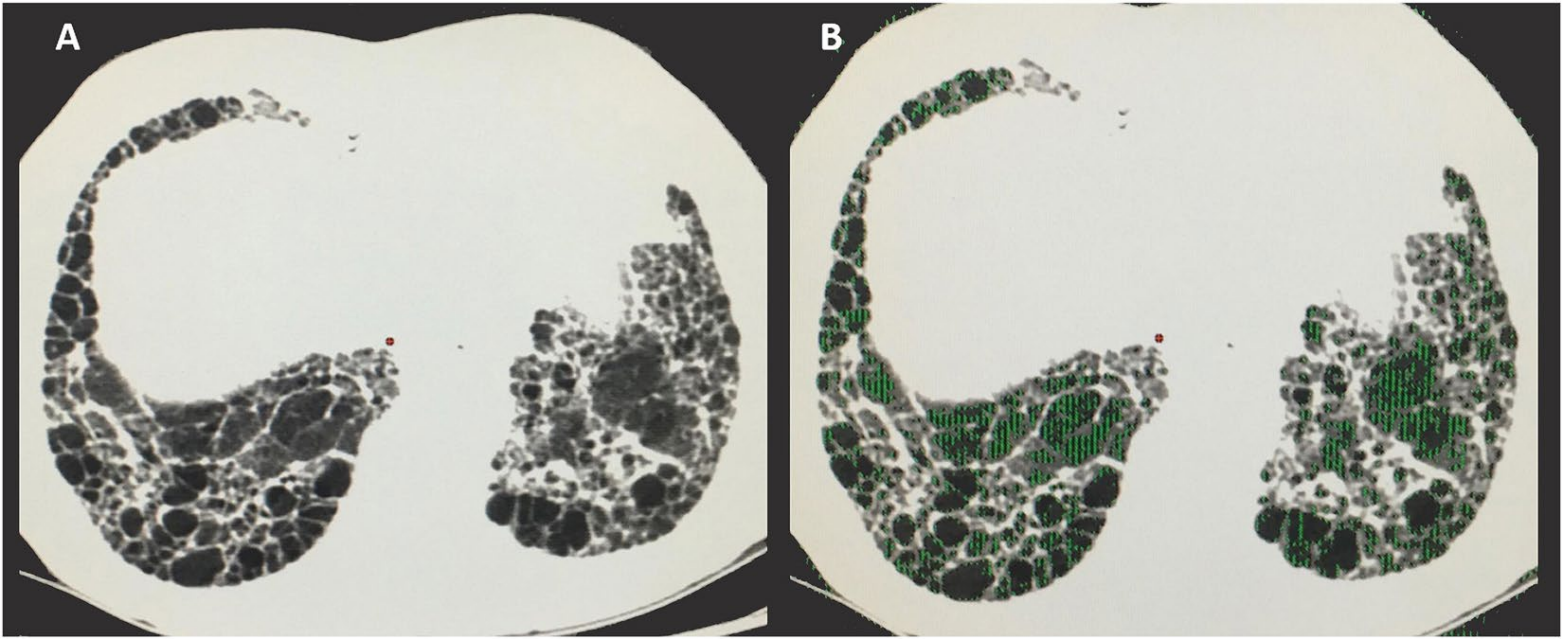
Expiratory CT from a subject with IPF associated with air trapping. Images from an 83-year-old patient diagnosed with IPF. (A) Axial chest CT scan demonstrates extensive areas of honeycombing in the cortical of the inferior lobes, suggestive of UIP pattern. (B) Same CT slice demonstrating the air trapping regions colored in green (ATIexp = 31.6%, E/I-ratio = 0.91).
